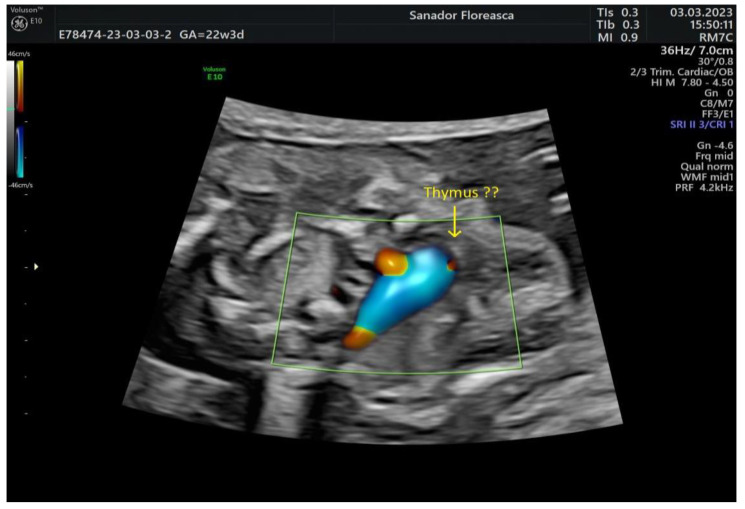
Three-vessel-trachea view in Color Doppler. Again, the continuity of the ascending aortic arch with the descending aorta cannot be demonstrated and the trachea is close to the dilated pulmonary artery. Note the presence of the hypoplastic thymus. ??: severe hypoplastic thymus.
